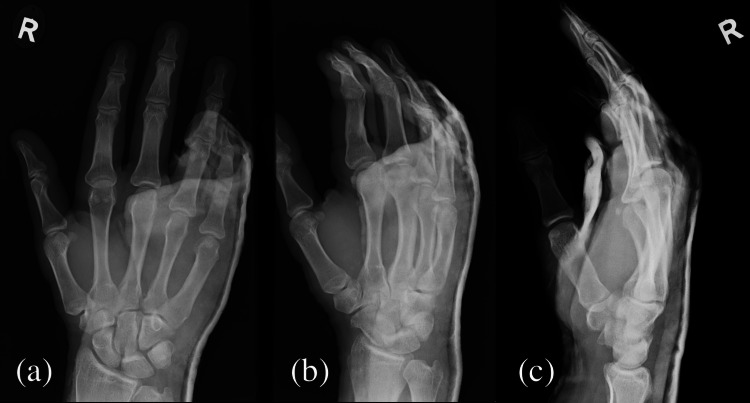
X-ray one-week post injury. (a) Anteroposterior view, (b) oblique view, and (c) lateral view.
